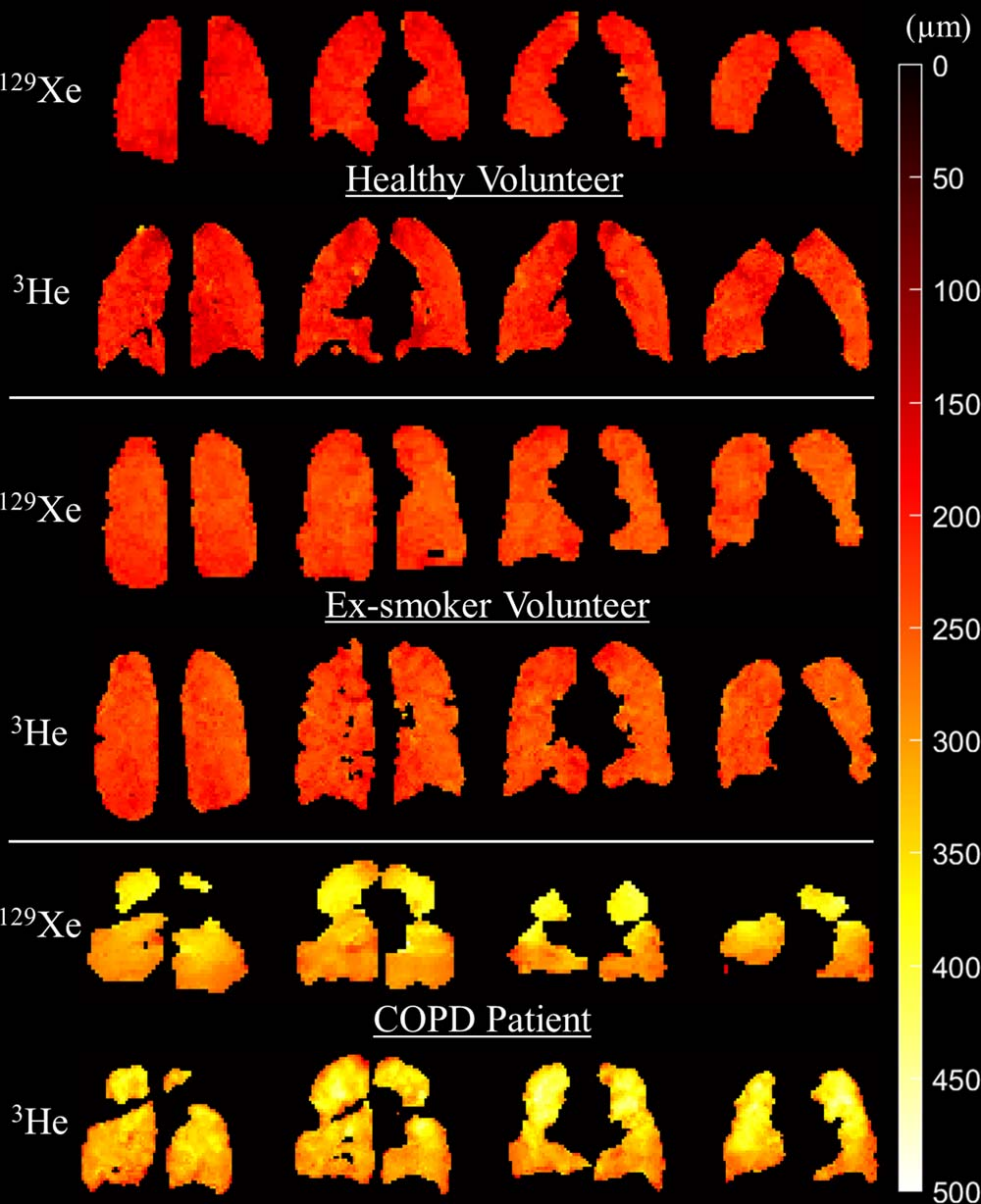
Comparison of ^129^Xe and ^3^He example slice Lm_D_ maps for a representative healthy, ex‐smoker, and COPD subject. ^129^Xe Lm_D_ maps derived using 3D multiple b‐value ^129^Xe DW‐MRI at an empirically optimized diffusion time Δ = 8.5 ms demonstrate good agreement with ^3^He Lm_D_ maps. 3D ^129^Xe DW‐MRI mean SNR was 37, 44, 80 for the representative healthy volunteer, ex‐smoker, and COPD patient, respectively.
